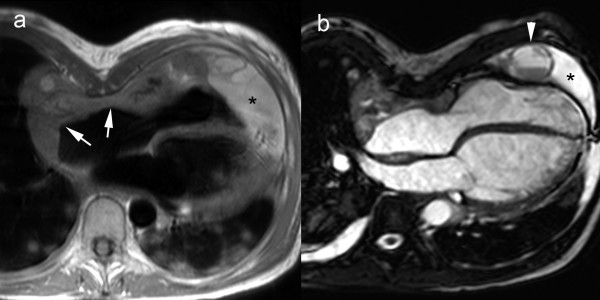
Cardiac angiosarcoma. Axial T1-weighted spin-echo CMR (a), axial cine CMR (b). Mass arising from right atrial lateral wall (arrows) extending to anterior atrioventricular groove and pericardium. Presence of a pericardial effusion (*) with several nodular masses (arrowhead). Note the presence of multiple pulmonary metastases. Presence of pectus excavatum and right-sided pleural effusion.
